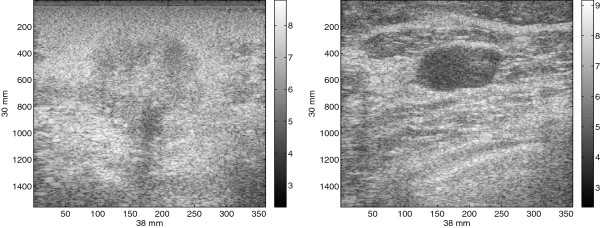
Uncropped B-mode ultrasound images of breast tissue, malignant tumor (left) and benign tumor (right).
